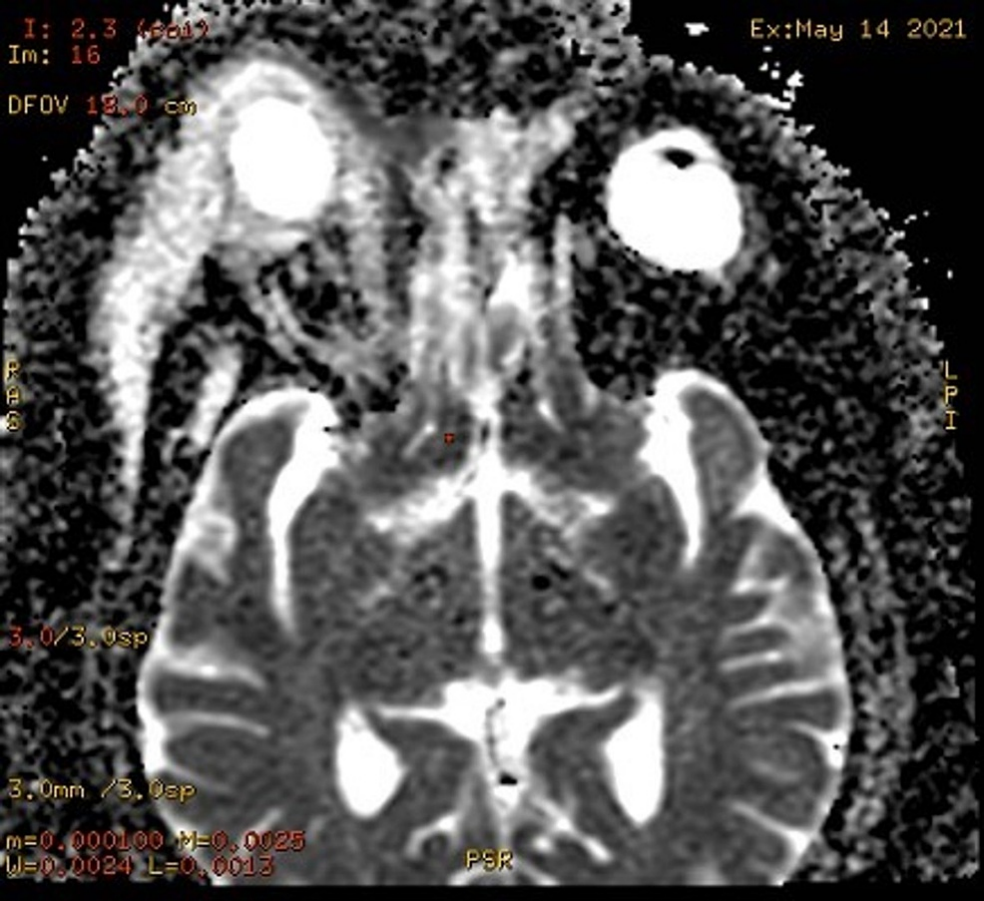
MRI DWI brain - sagittal view with contrast The image shows heterogeneous enhancement in the pre-septal orbit around the sclera with mild mucosal enhancement in the right maxillary and ethmoidal sinuses MRI: magnetic resonance imaging; DWI: diffusion-weighted imaging
